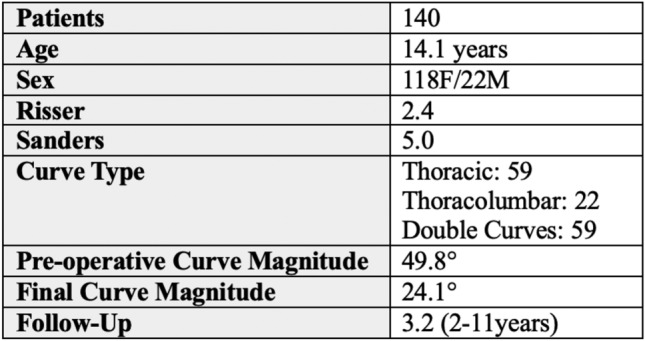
Demographic and radiographic data for 140 AIS patients with ≥ 2 year follow-up stratified by curve location and the number of curves treated
